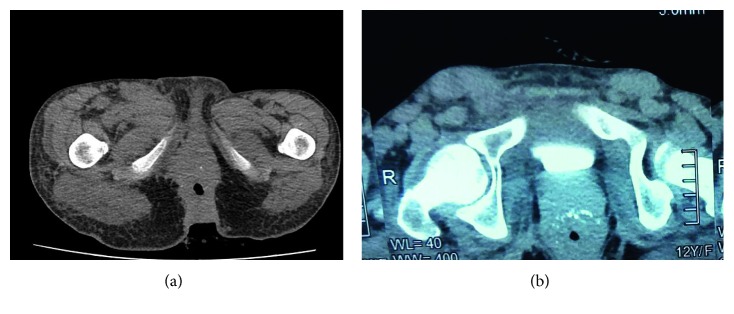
Anal and rectocanal thickening with inguinal and iliac lymph node involvement on CT scan.
